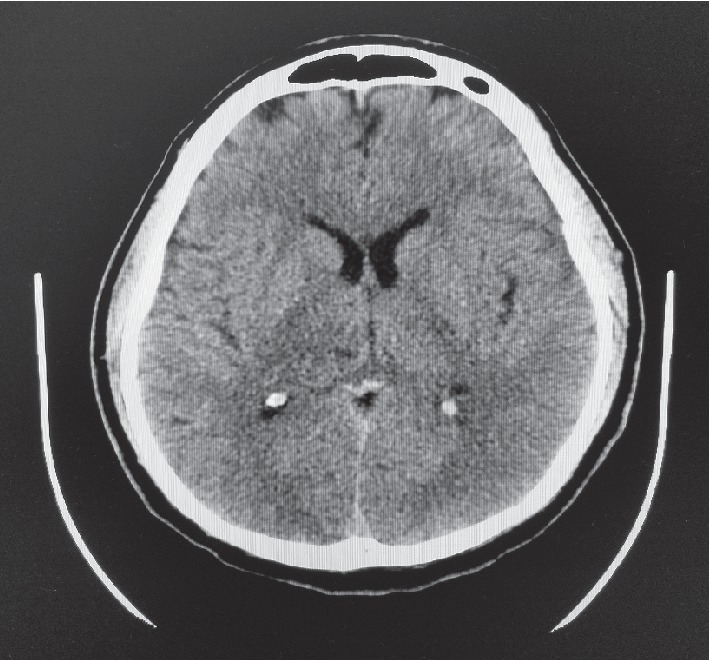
CT brain without contrast interpreted as normal.
